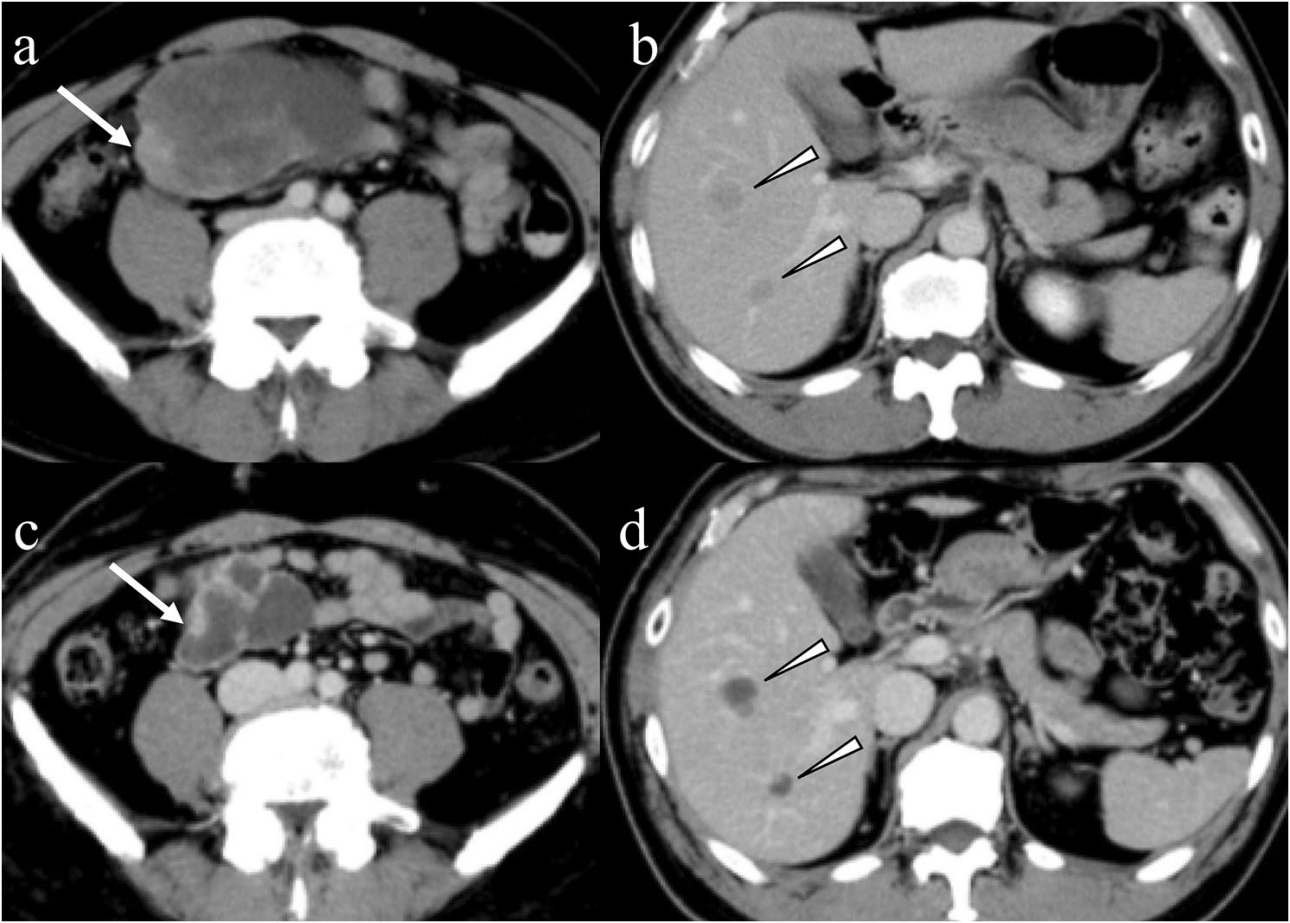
Imaging findings after imatinib therapy. A 54-year-old male with high-risk gastrointestinal stromal tumor in the small bowel was treated with imatinib. A bulky tumor with degeneration (a: arrows) and hepatic metastases (b: arrowheads) are seen in the venous phase of contrast-enhanced CT. The primary small bowel lesion decreased in size (c: arrow). Hepatic metastases show no change in size, whereas the contrast enhancement markedly decreased (d: arrowheads)
